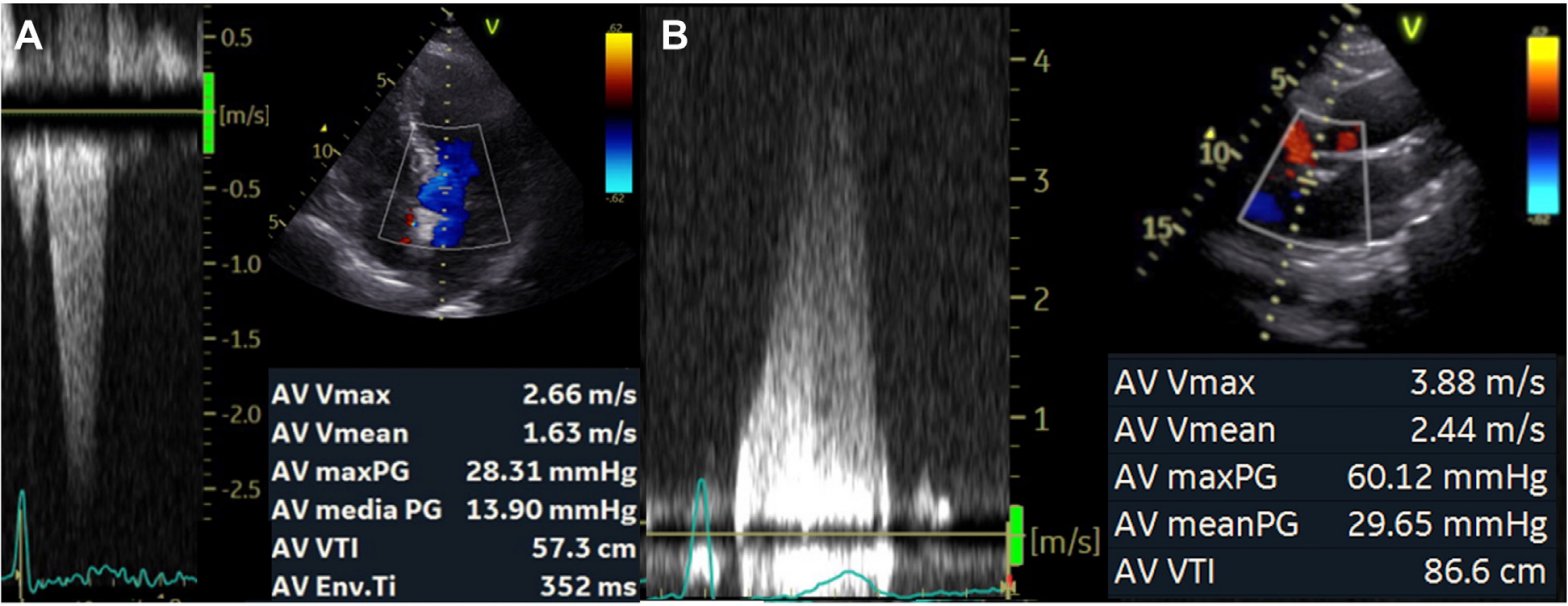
Left Ventricular Outflow Tract Obstruction Left ventricular outflow tract obstruction as assessed from the apical (A) and right parasternal (B) views.
